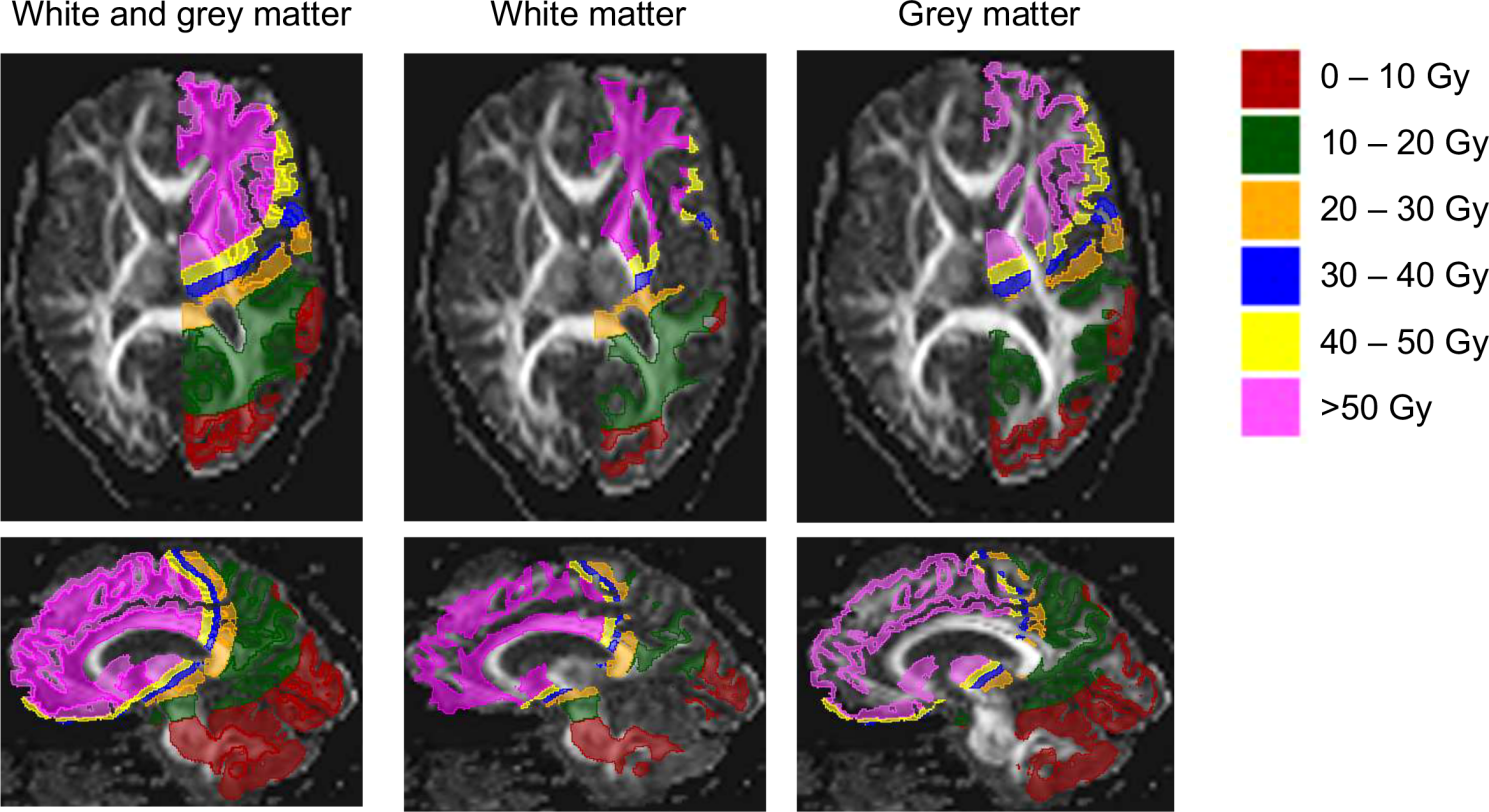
Segmentation shown for grey and white matter projected on fractional anisotropy (FA) maps. Images shown are obtained in a patient aged 51 years at RT onset, diagnosed with an oligodendroglioma in the right frontal lobe (not shown), and 46 months between the end of RT and the MRI scan.
